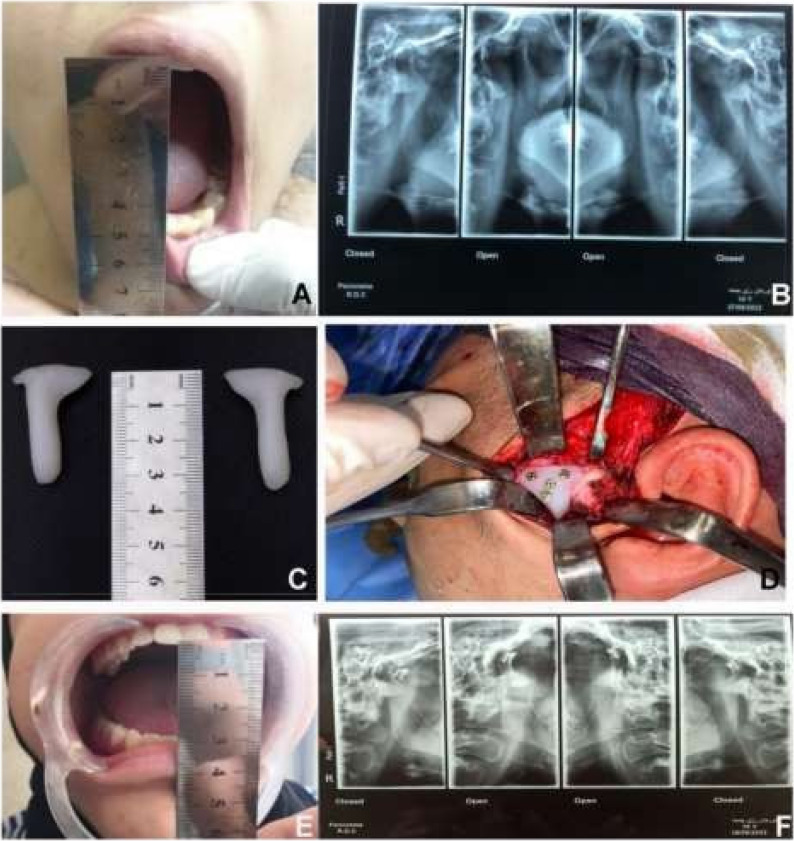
A Preoperative MIO about 45mm (B) preoperative x ray TMJ open and closed (C) custom-made UHMWPE implant (D) Intraoperative after placement of the custom-made UHMWPE implant (E) Postoperative MIO about 30mm (F) Postoperative X-ray TMJ open and closed
